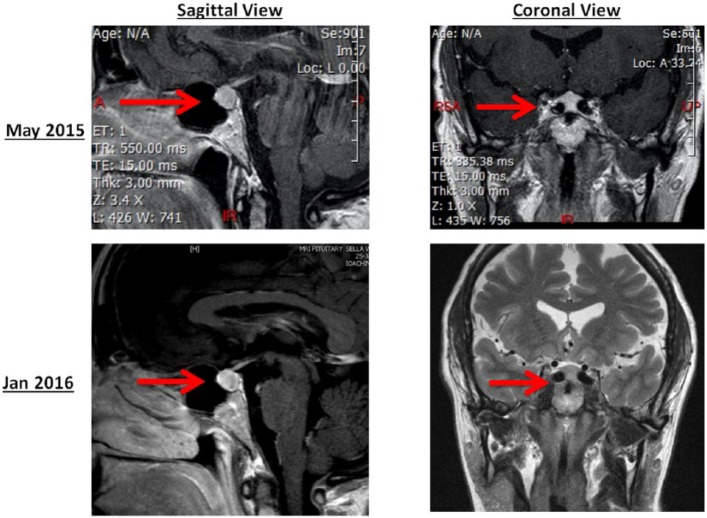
MRI of the pituitary gland at presentation (May 2015) and after restoring euthyroidism (Jan 2016).
